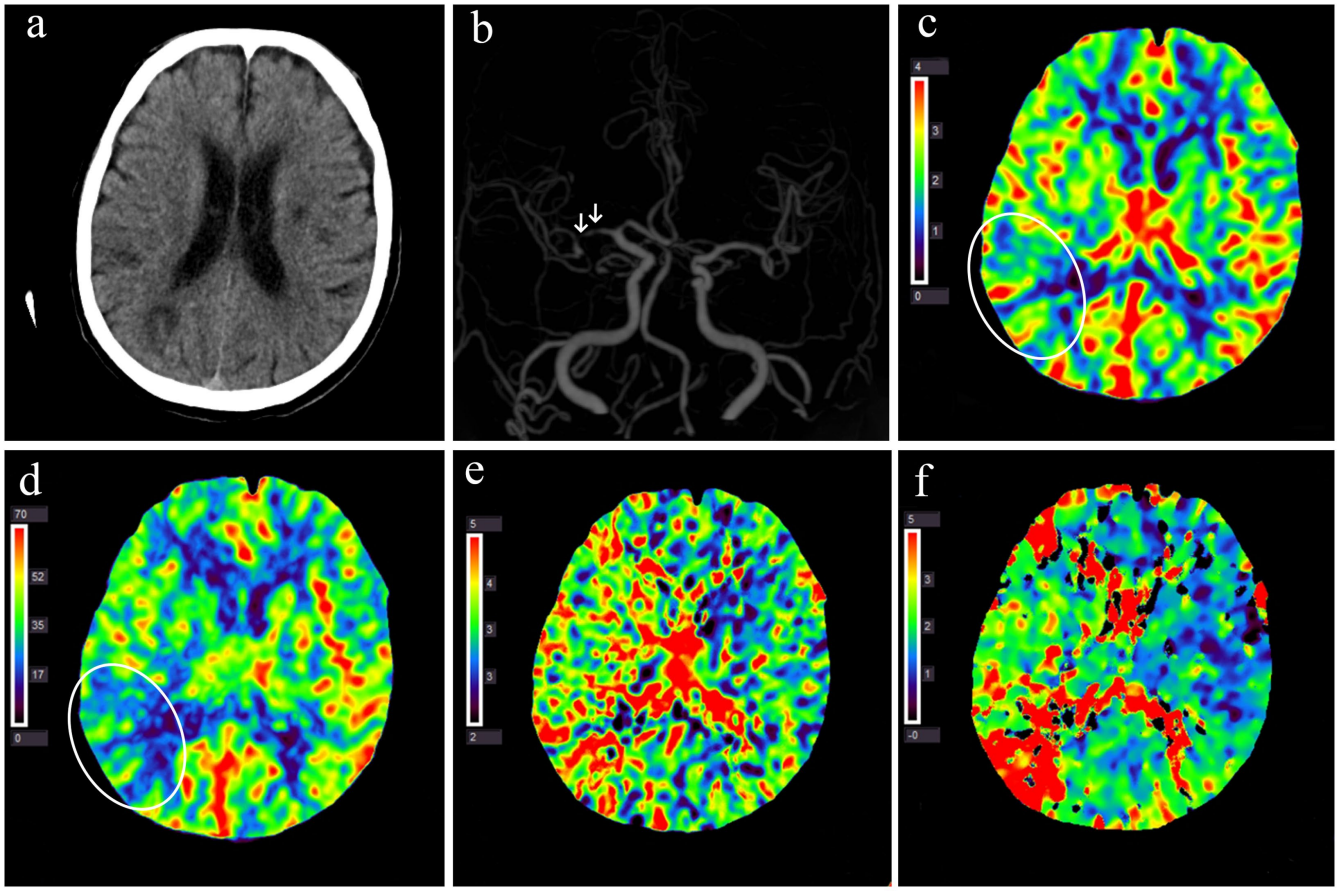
Multimodal CT examination of a patient with acute infarction at the right occipitoparietal junction 1 day after symptom onset. (a) Non-contrast CT shows multiple hypodense lesions in the right parieto-occipital lobe and bilateral basal ganglia. (b) CT angiography reveals multiple severe stenoses in the M1 segment of the right middle cerebral artery (white arrow). (c–f) Perfusion imaging demonstrates decreased CBV (c) and CBF (d) in the right occipitoparietal junction (white circles) and prolonged MTT (e) Tmax (f) in the right hemisphere. CBF, cerebral blood flow; CBV, cerebral blood volume; MTT, mean transit time; Tmax, the time to the maximum of residue function.
